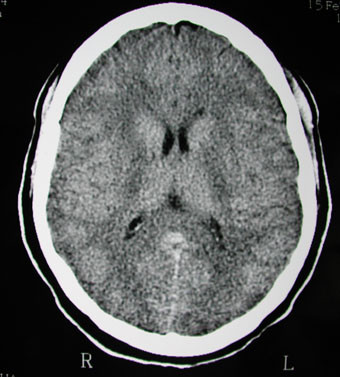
CT scan showing diffuse cerebral edema in a young woman with hypersensitivity syndrome due to minocycline.
